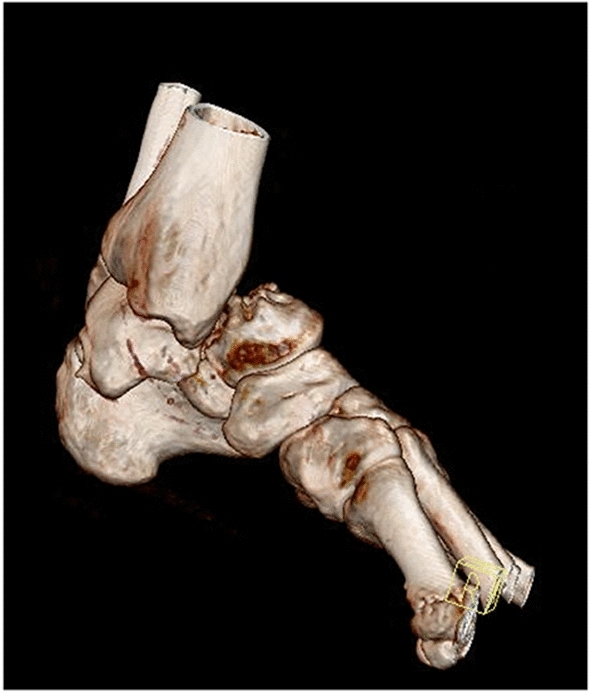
CT three-dimensional reconstructed sagittal image of a left sided displaced navicular and cuboid fracture with talo-calcaneal dislocation
